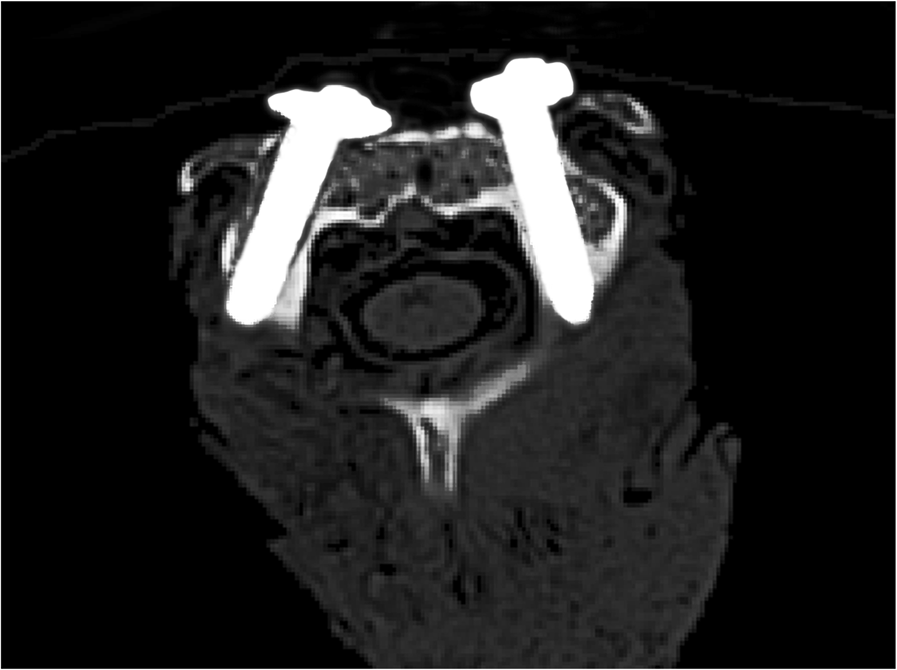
Postoperative CT scan showing good positioning of the pedicle screws (axial view)
